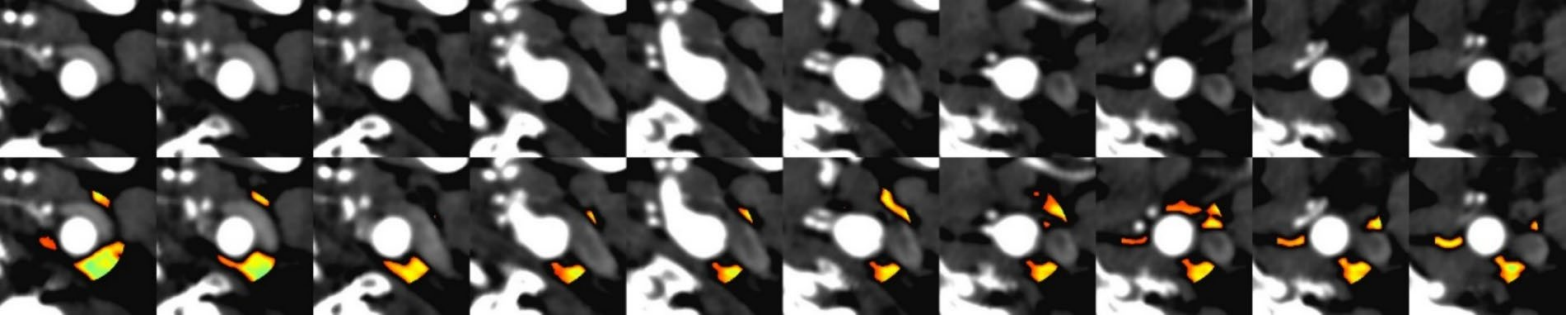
Comparison of CT angiography (CTA) imaging and automated perivascular fat density extraction: (A) Upper row: Conventional CTA cross-sectional images. (B) Lower row: Corresponding images with automated fat density extraction, where color-coded regions indicate perivascular adipose tissue (PVAT) distribution.
